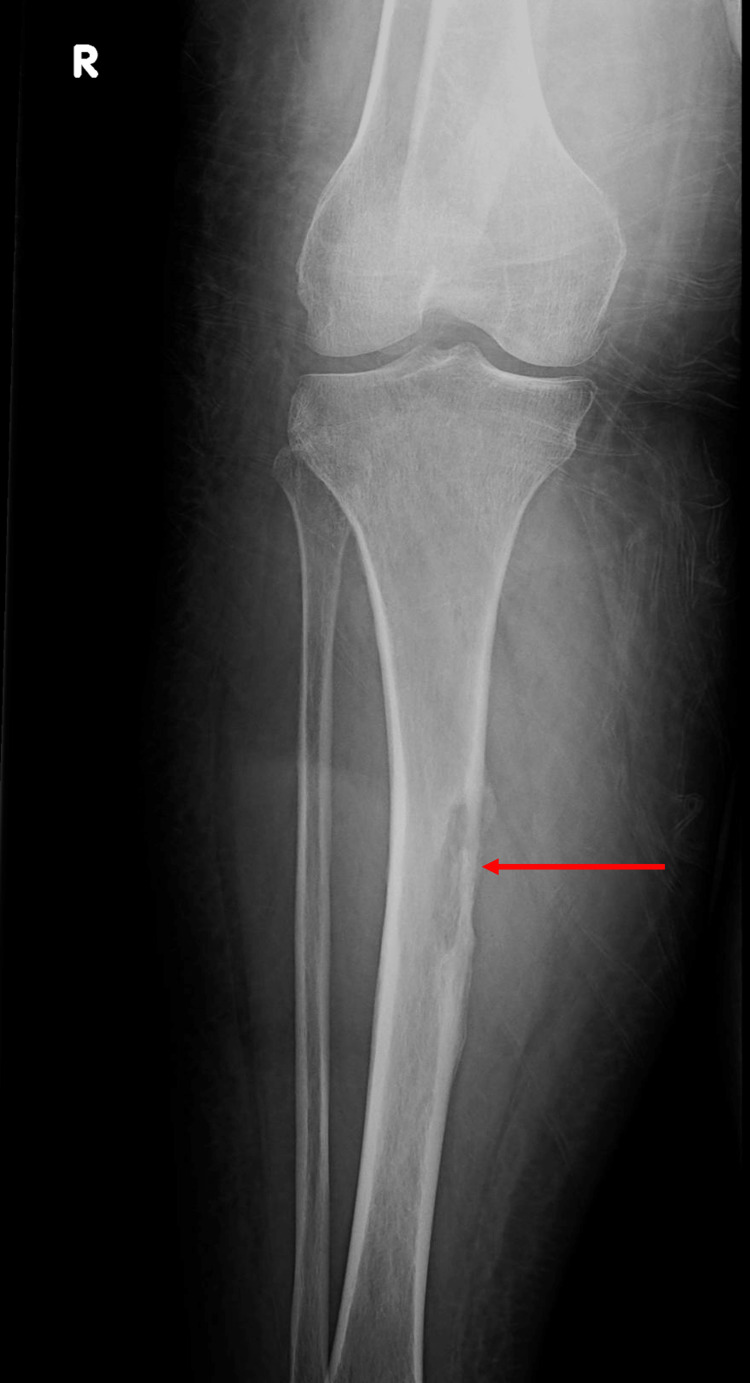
Anteroposterior radiograph of the right knee demonstrating an incidentally found lucent lesion with adjacent periosteal reaction within the proximal tibial diaphysis (red arrow).
